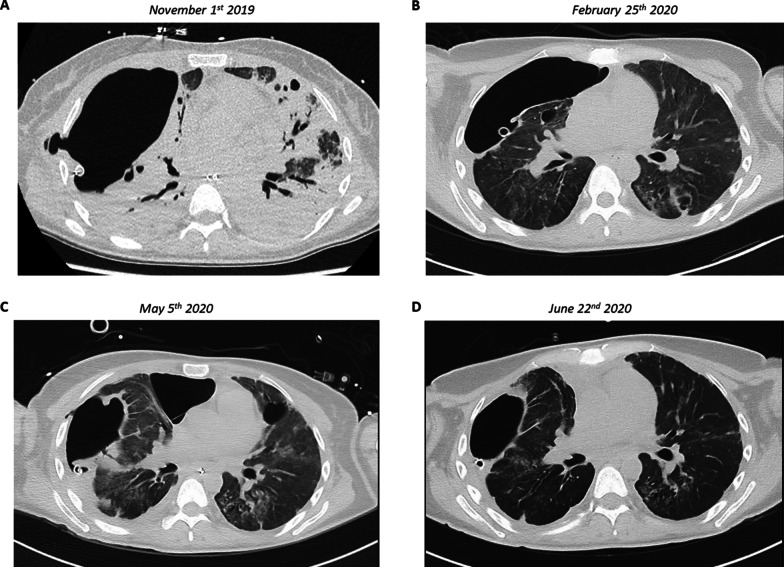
Consecutive CT images showing subsequent phases in the disease course. a
